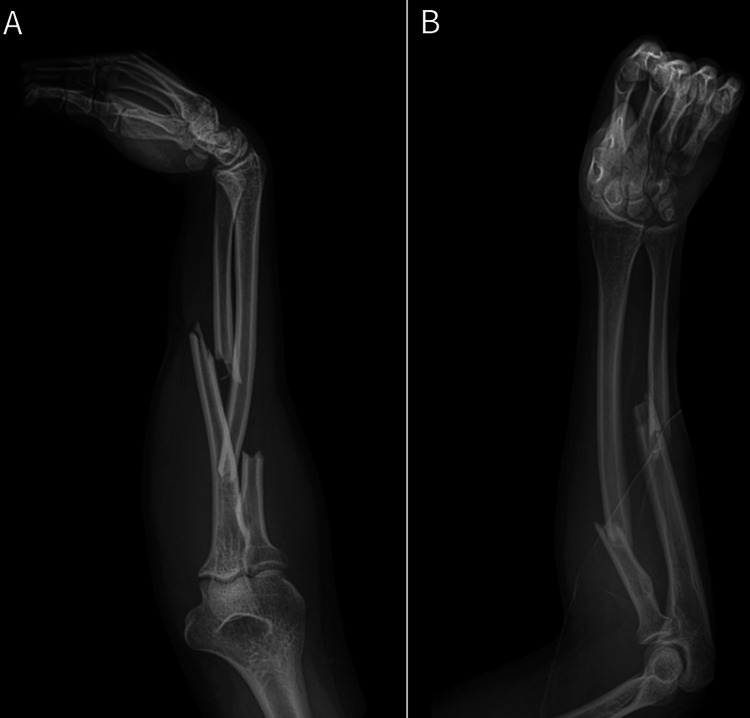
Pre-operative radiographs A) Lateral forearm radiograph; B) anteroposterior forearm radiograph
